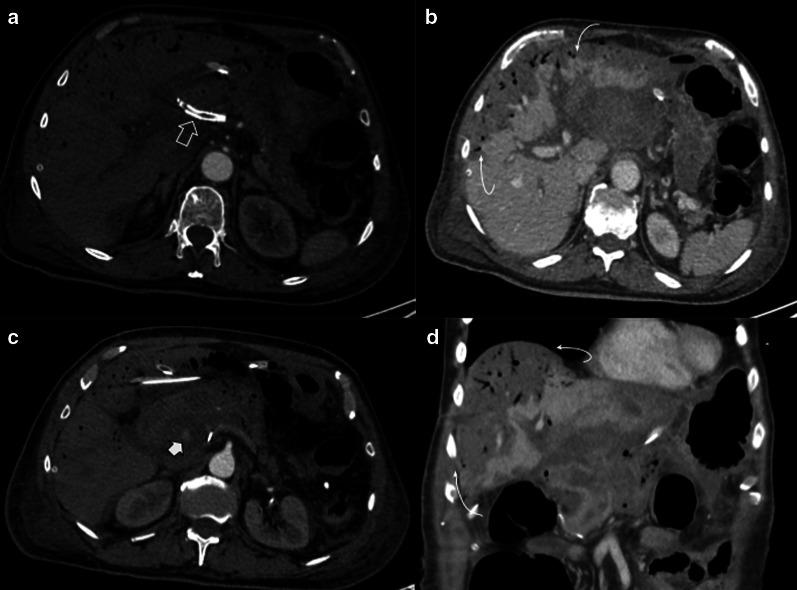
Hepatic infarct. 78-year-old patient with complicated early postoperative due to pseudoaneurysm. Stent in the hepatic artery was placed. Control CT scan due to increased hematic fluid drainage evidence occluded stent (blank arrow in a) and big subcapsular hypodense areas in liver parenchyma (curved arrows in b and d) consisting with infarct. An active bleeding focus depending on the ligated gastroduodenal branch was also detected (big arrow in c)
